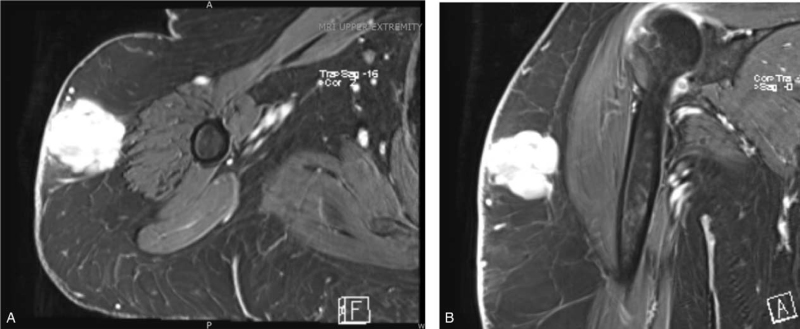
Initial presentation MRI A) axial, volumetric interpolated breath-hold examination (VIBE) sequence with gadolinium contrast; B) coronal VIBE sequence with gadolinium contrast.
